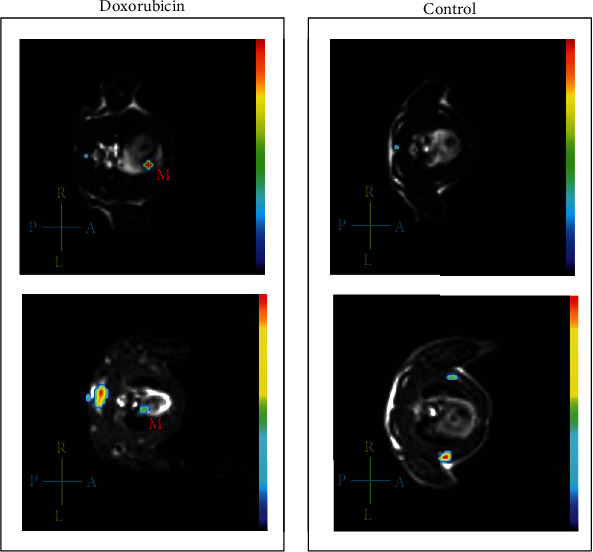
^19^F signal (color overlay) over the ^1^H anatomical scan in 1.5 Tesla MRI (black and white). The red label “M” marks the myocardial fluorine signal. All other displayed signals are attributed to enhancement in the lymph nodes, especially in the dorsal thoracic region, where a large collection of lymph nodes near the shoulder blades is located [21].
